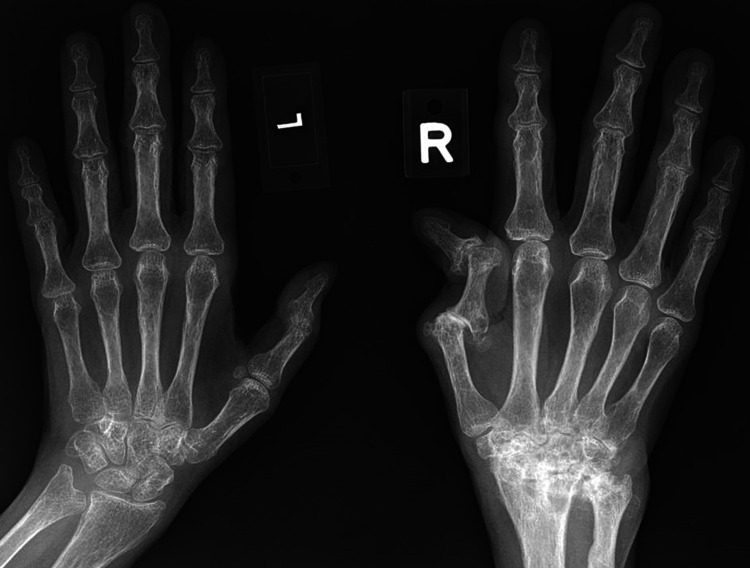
X-ray of the hands, PA view Advanced degeneration of the right wrist with the collapse of the carpal bones with boutonniere deformity of the right thumb while the left hand was unremarkable - save for osteopenia
